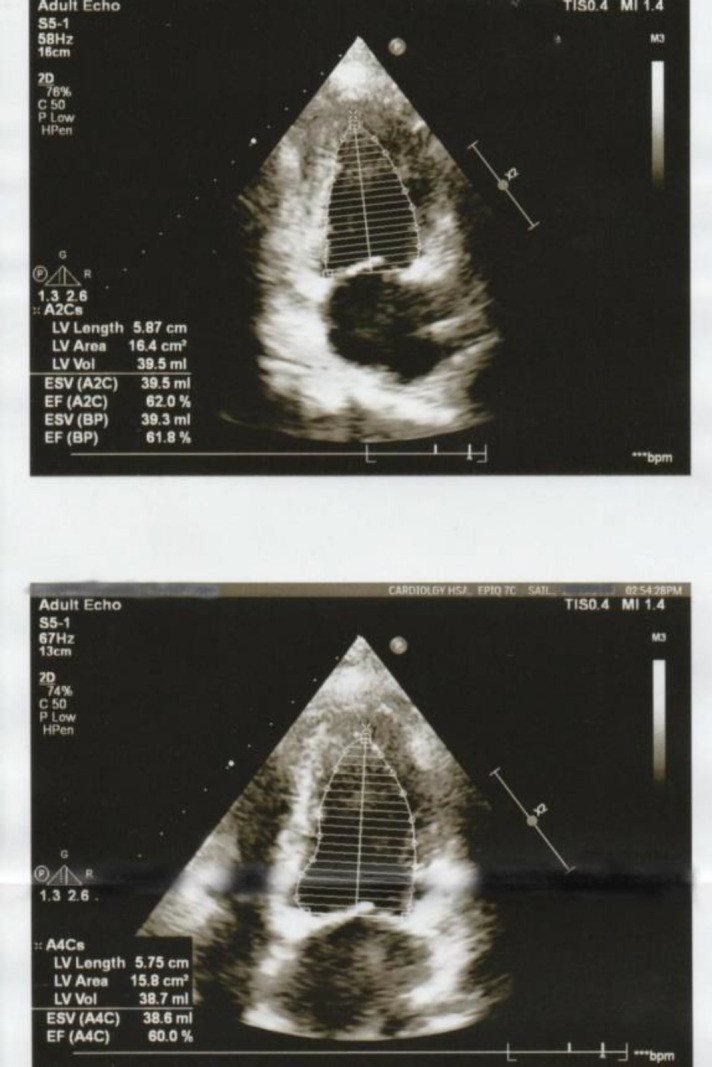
Modified Simpson's biplane method for measurement of ejection fraction in the apical two-chamber and four-chamber view
